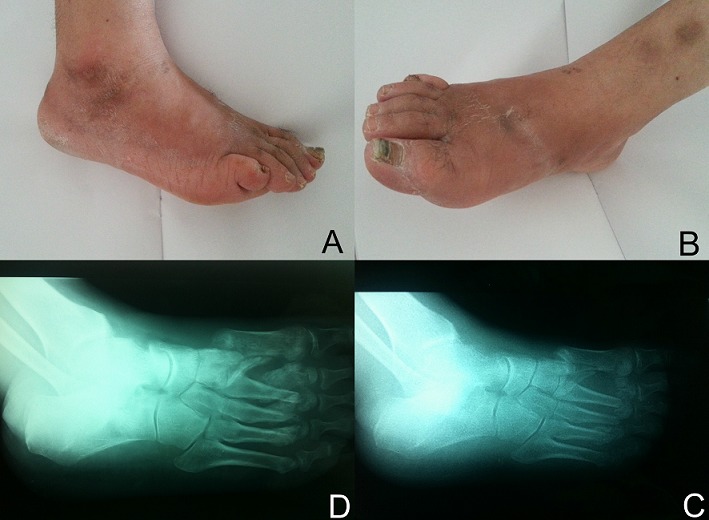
A) photo de profil du pied droit; B): photo de face du dos du pied droit; C): radiographie de profil du pied droit montrant des fractures des têtes des métatarsiens-2^ème^, 3^ème^ et 4^ème^ rayons et une lyse osseuse de la tête et de la base du 1er métatarsien; D) radiographie de profil du pied droit montrant des fractures déplacées, des luxations articulaires et des géodes sous-chondrales des têtes métatarsiennes
